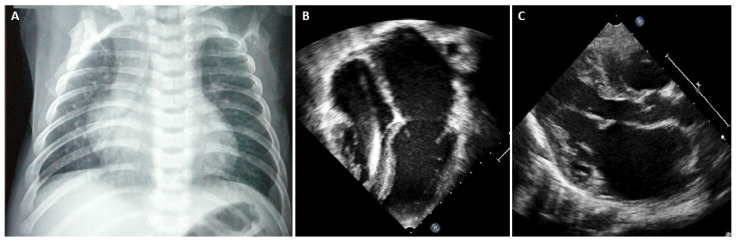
(A) Chest X-ray, 8-year-old boy with hypertrophic-restrictive cardiomyopathy and congestive heart failure. (B) Echocardiography apical 4-chambers view, 11-year-old girl with restrictive cardiomyopathy, normal ventricular volumes, preserved ejection fraction and severe atrial dilatation; we can appreciate the presence of an implantable cardiac defibrillator lead. (C) Echocardiography parasternal long axis view, 6-year-old boy with restrictive cardiomyopathy associated to severe left atrial dilatation.
